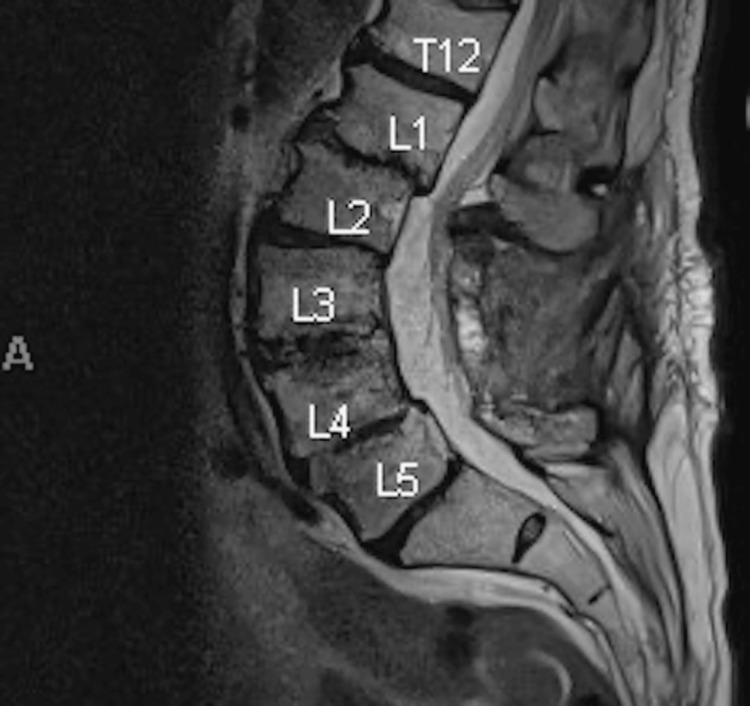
MRI spine of case 1 Soft tissue edema of the laminectomy bed and tiny enhancing area of fluid
